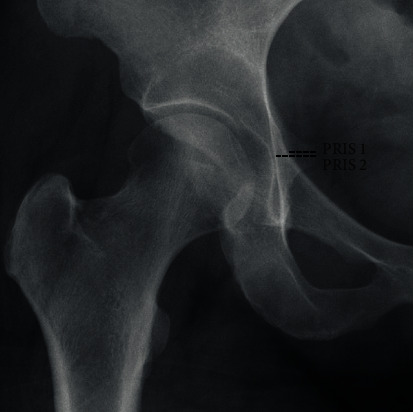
Pelvic AP radiograph showing PRIS 1 and PRIS 2 measurements.
